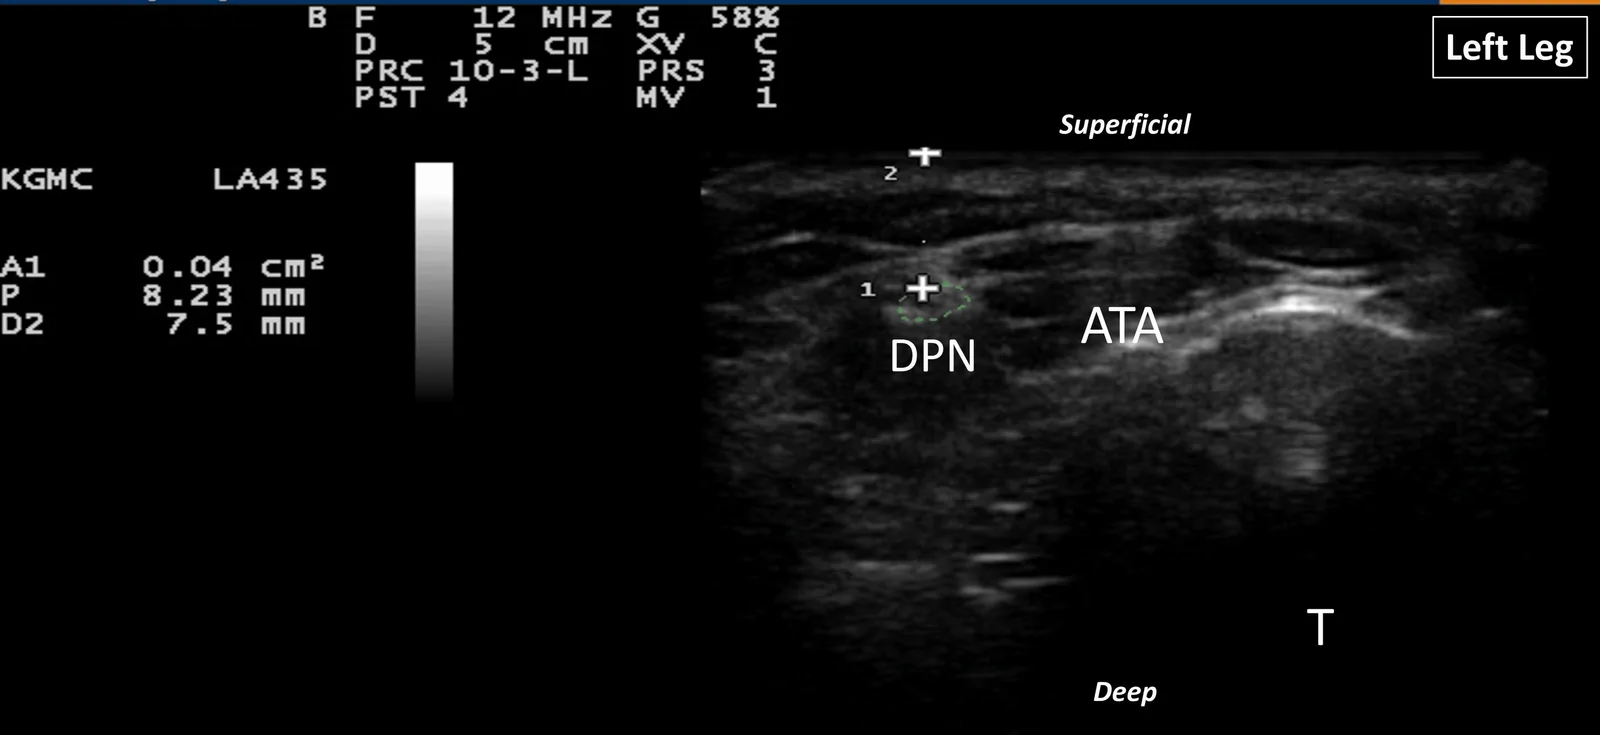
Ultrasonographic image of left leg showing the deep peroneal nerve at 3 cm above the superior extensor retinaculum. TA, anterior tibial artery; DPN, deep peroneal nerve; T, tibia.
